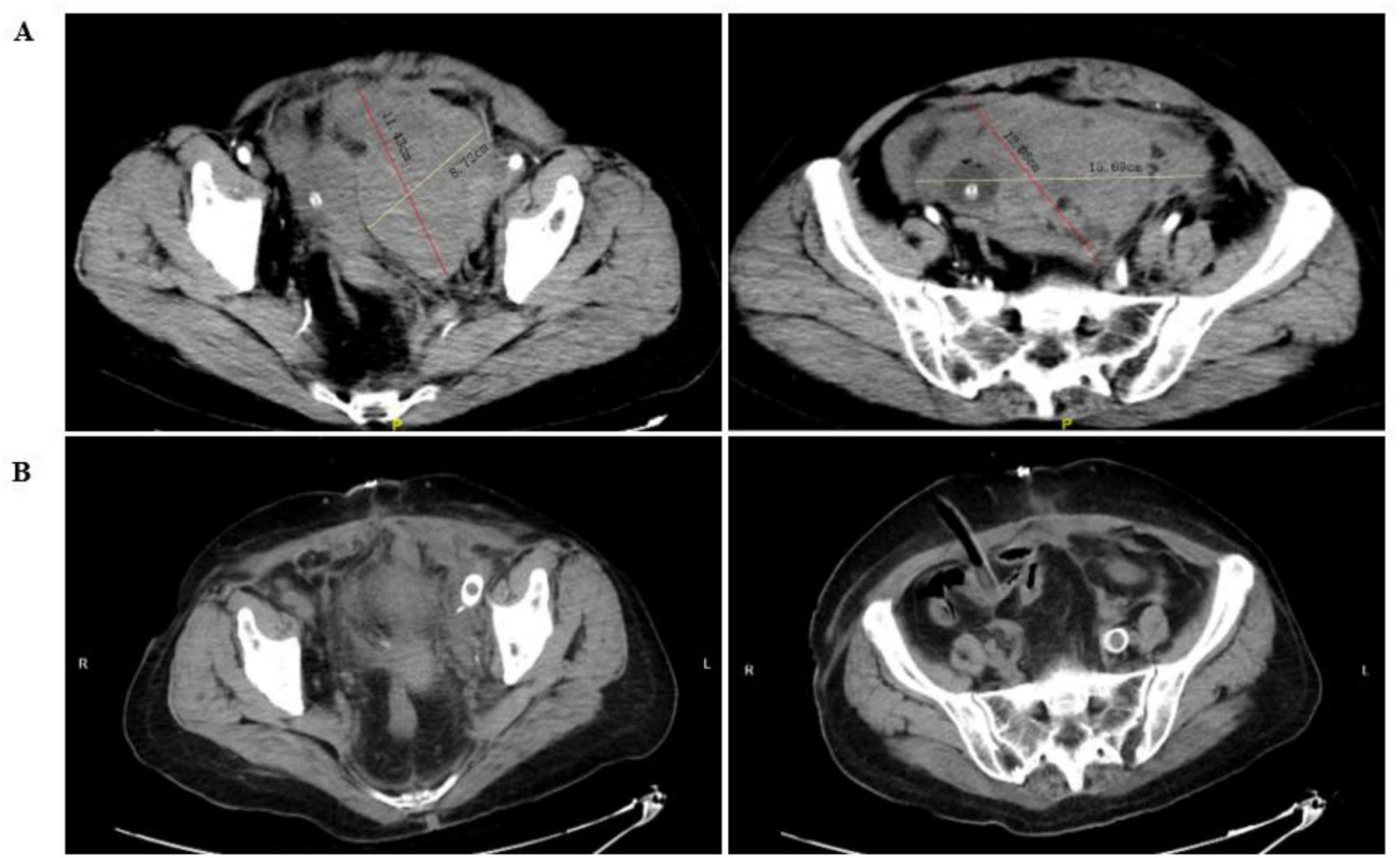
The CT examination image of the patient. (A) Shows the image before the surgery, and (B) shows the image after the surgery.
